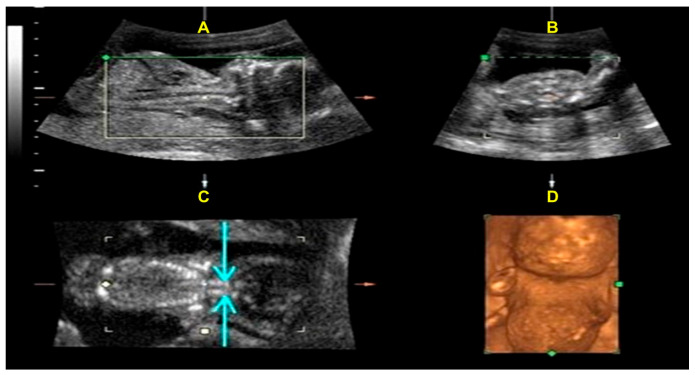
The coronal view of the fetal neck on 3D ultrasound showed absent ossification of the vertebral bodies (the light blue arrows in the left lower figure indicate only ossification of vertebral pedicles) (C); the 3D ultrasound revealed a flat face, micrognathia (A,D), and a small and narrow chest (B,D).
